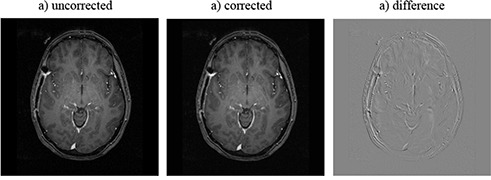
A sample MR image slice of a radiation therapy patient's brain (patient was diagnosed with GBM): a) raw image, b) same image slice corrected using the distortion map determined using our procedure, and c) the difference of the two.
